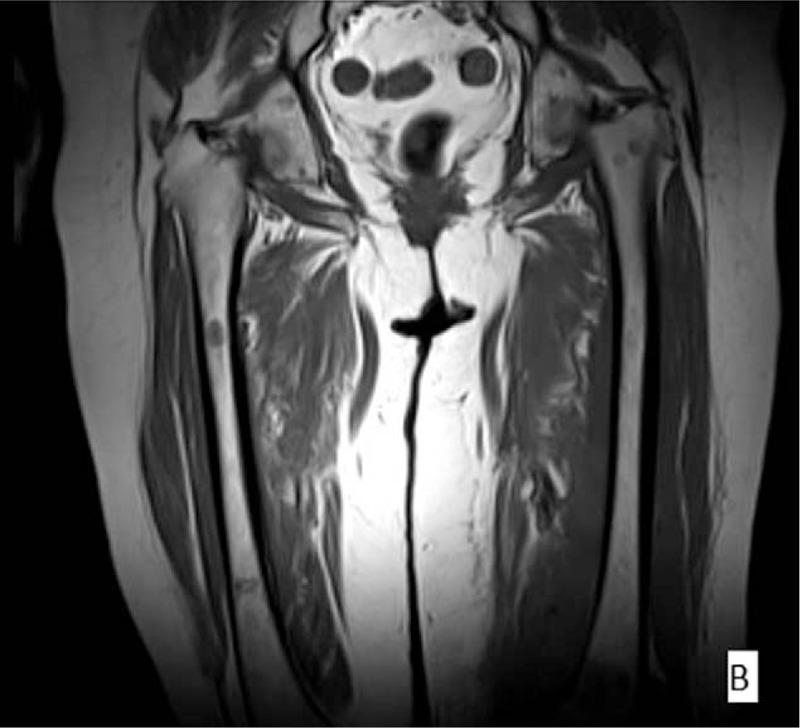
Coronal T1-weighted image.^[4]^
